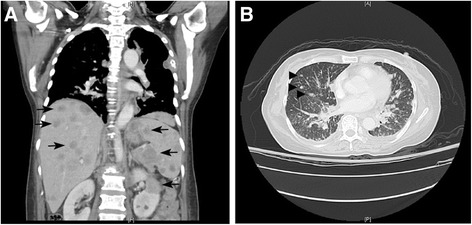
Chest and abdominal computed tomography (CT) of the patient. (A) Multiple liver, spleen, and left kidney metastases (arrow). (B) Lung nodules favor metastases (arrowhead).
